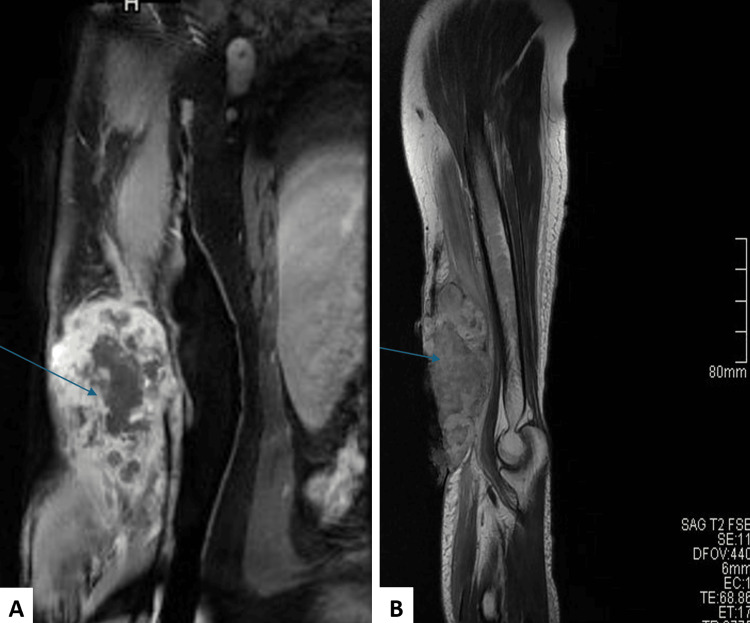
Imaging showing the coronal MRI of the right arm highlighting the heterogeneous, lobulated tumor mass (A) and the sagittal T2-weighted MRI demonstrating tumoral invasion and vascularization (B). Arrow in (A): the tumor is seen involving the anterior aspect of the distal right arm with skin ulceration and exposure of tumoral tissue. Arrow in (B): the tumor is shown invading the biceps brachialis, brachialis, and anteromedial brachioradialis with vascularization via the radial collateral artery.
